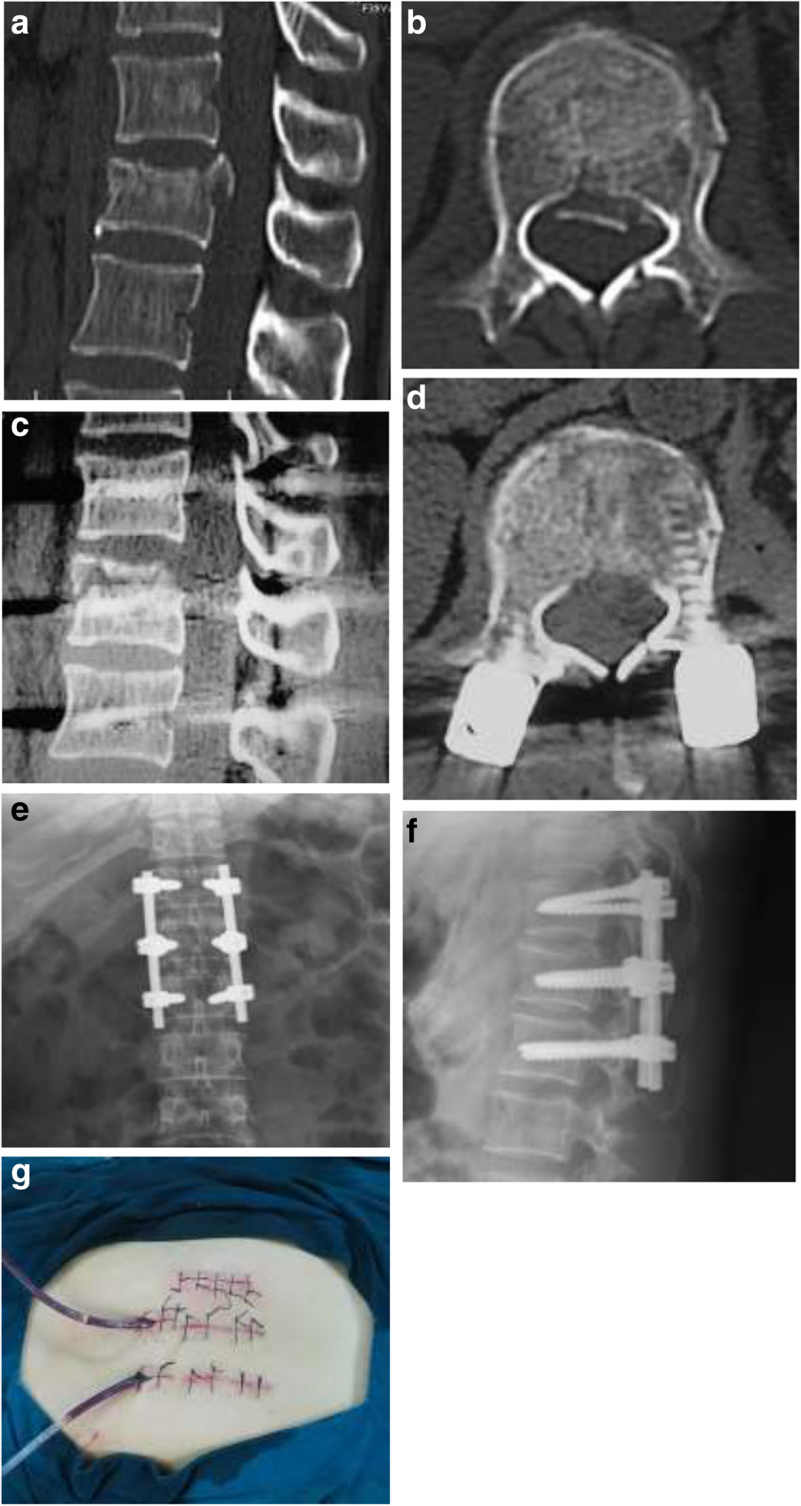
Images obtained in a 38-year-old woman who underwent direct decompression followed by percutaneous short-segment fixation: (a) Preoperative sagittal sectional CT; (b) Preoperative cross-sectional CT; (c) Postoperative sagittal-sectional CT; (d) Postoperative cross-sectional CT; (e) Postoperative anteroposterior X-ray; (f) Postoperative lateral X-ray; (g) Longitudinal incision next to erector spinae.
